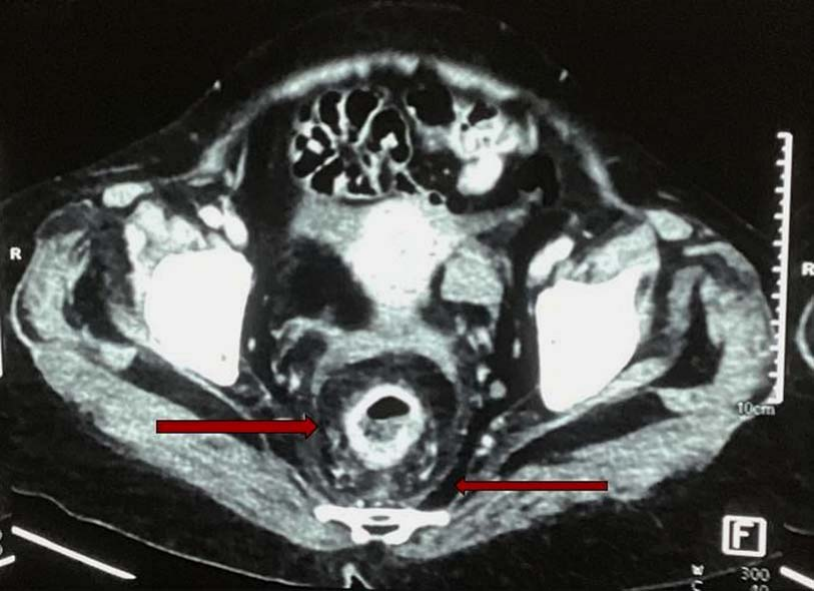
Contrast-enhanced computed tomography abdomen and pelvis showing homogeneous enhancing circumferential thickening in rectum with surrounding fat strandings (red arrows).
